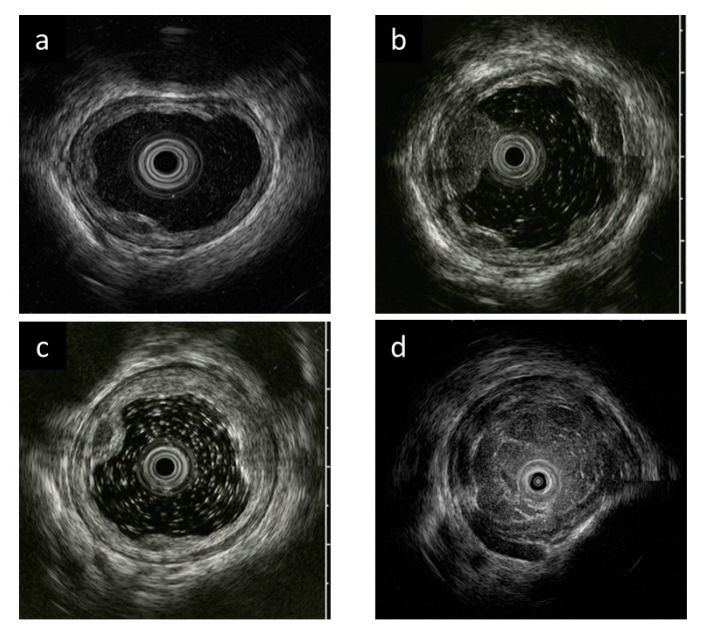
Evaluation criteria for endoscopic ultrasonography (EUS) image quality. (a) Excellent (4 points). Clear images with no debris-like artifacts in EUS. (b) Good (3 points). A few debris-like artifacts are present, but do not interfere with the evaluation of esophageal varices (EVs) in EUS. (c) Moderate (2 points). Debris-like artifacts are noticeable, but do not interfere with EV evaluation in EUS. (d) Poor (1 point). Difficult to assess EV due to debris-like artifacts and poor esophageal lumen expansion in EUS.
